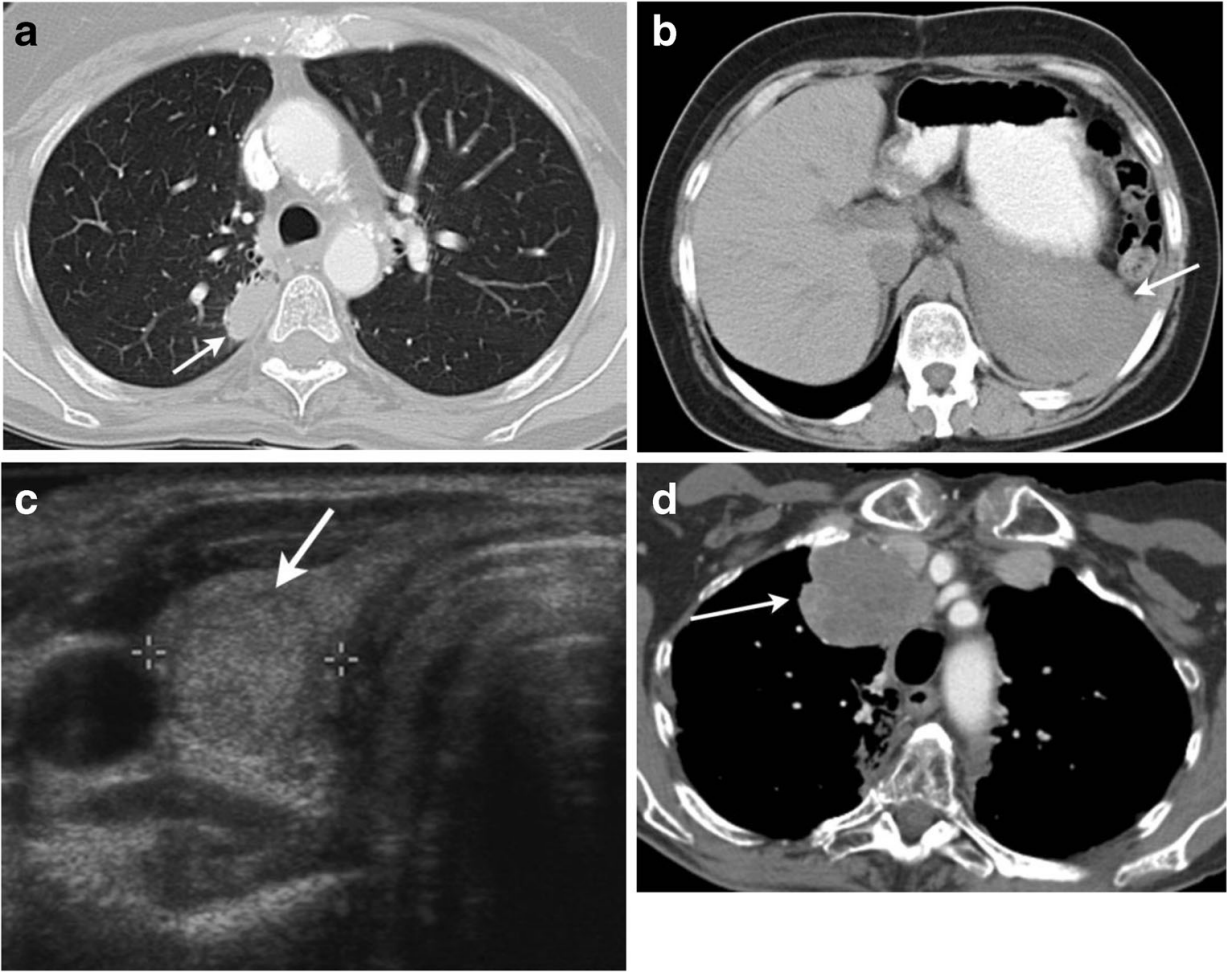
Second malignancies in patients with HL. a A 40-year-old female with a history of nodular sclerosing HL at the age of 15 treated with chemotherapy and upper mantle radiation. Axial contrast-enhanced lung window CT image shows a soft tissue mass within the right paramediastinal radiation field. Pathology was suggestive of adenocarcinoma of the lung. b A 24-year-old female presenting with upper abdominal pain and fullness with a prior history of HL at the age of 13 treated with splenectomy and radiation. Axial non-enhanced CT image of the upper abdomen shows a soft tissue mass lesion in the left upper abdomen (*arrow*). Pathology was suggestive of mesothelioma. c A 48-year-old female with a history of nodular sclerosing HL at the age of 15 treated with chemotherapy, mantle and pelvic radiation. Transverse greyscale ultrasound image shows a solid nodule in the right lobe of the thyroid (*arrow*). Fine-needle aspiration cytology from the nodule was suggestive of papillary thyroid carcinoma. d A 56-year-old female presenting with shortness of breath, intermittent cough and weight loss with a prior history of HL treated with radiation 40 years ago. Axial contrast-enhanced CT image shows a large heterogeneously enhancing anterior mediastinal mass (*arrow*). Pathology was suggestive of radiation-induced sarcoma
